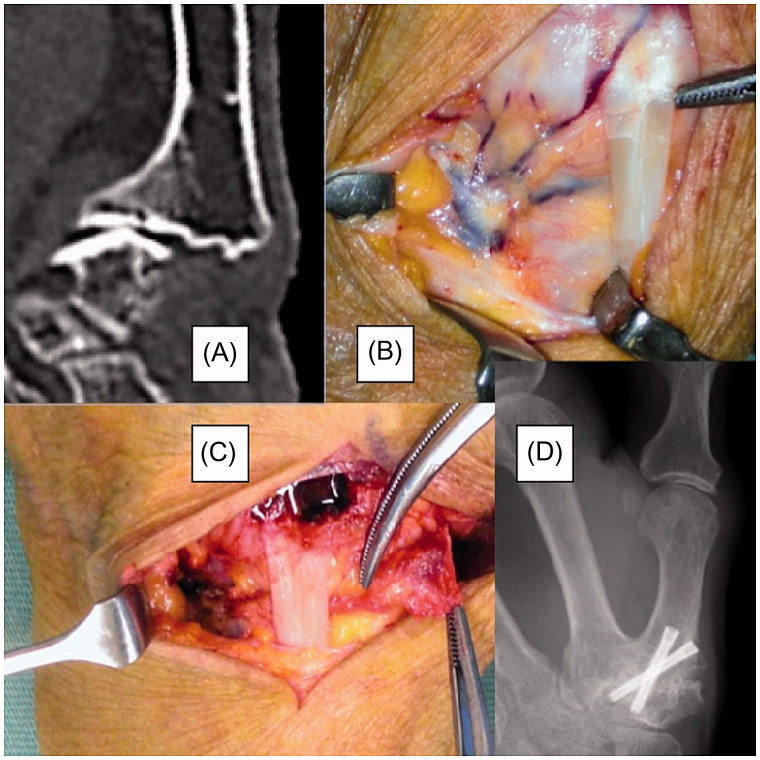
Case 2: a 78-year-old female. (A) Preoperative computed tomography showing thumb carpometacarpal arthritis with marked osteoporosis. (B) Intraoperative photograph of the second metacarpal artery. (C) Photograph of the vascularized bone graft at the time of transferal to the first carpometacarpal joint. (D) Radiography at 2 months postoperatively confirmed bony union.
